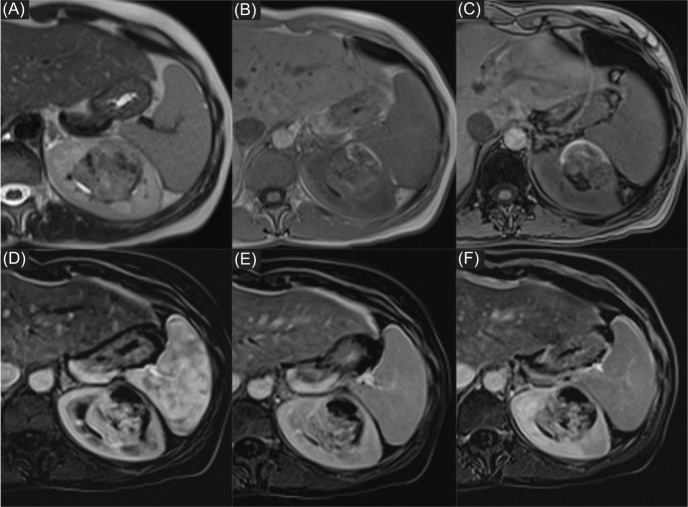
Abdominal MRI demonstrated the presence of an expansive capsulated heterogeneous mass, with inhomogeneous low-to-high signal intensity on (A) T2-weighted images; (B) partial high-signal intensity on in-phase image; and (C) on opposed phase image due to blood content partly and foci of internal fat. (D–F) Subtracted images confirmed an enhancing component in mid-internal portion of mass.
